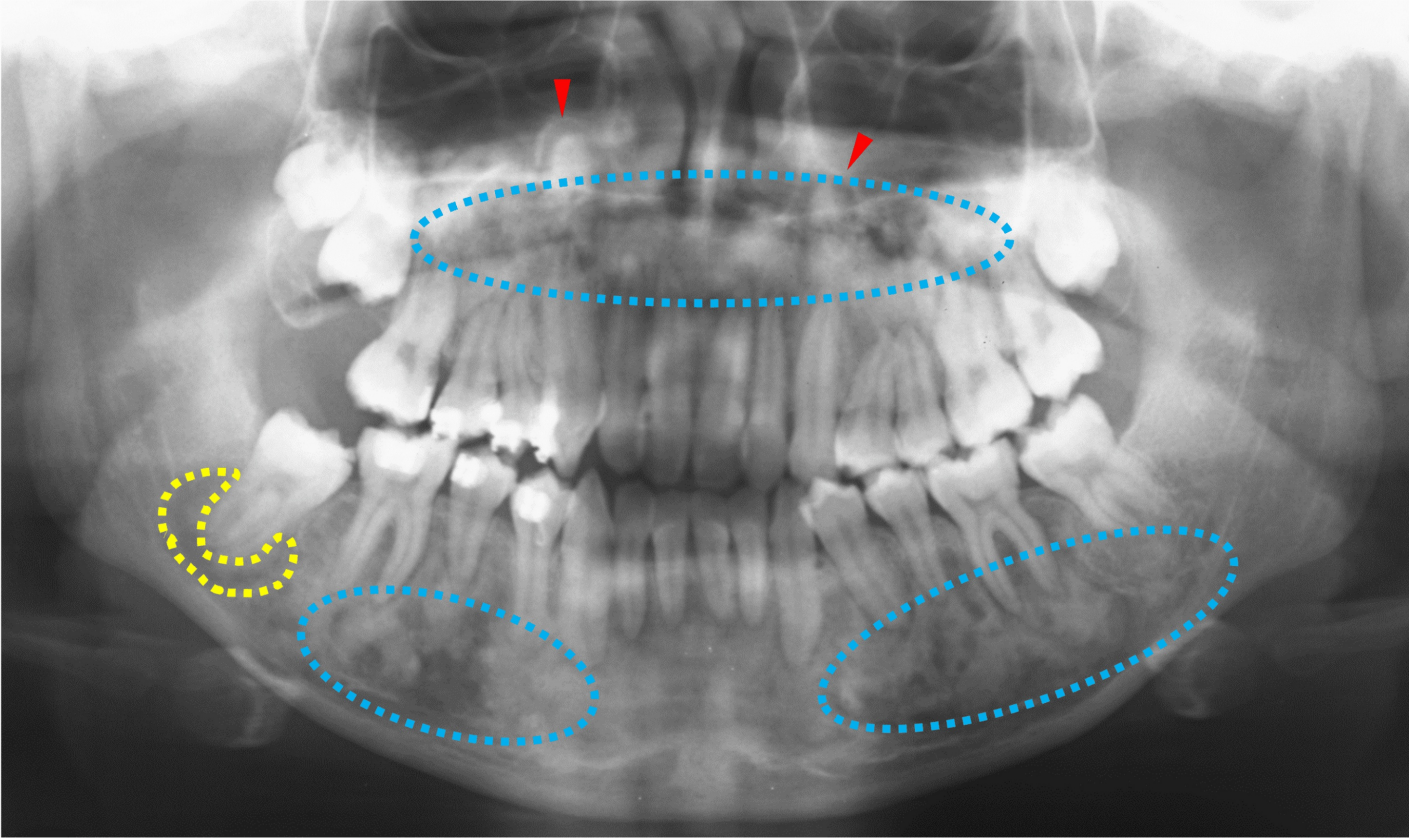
Maxillofacial radiograph at age 15 Blue dotted circles indicate the lesions in both the upper and lower jaws with enlarged frosted shadows. Red arrows indicate stenosis of the maxillary sinus. The yellow dotted circle indicates a radiolucent lesion adjacent to the right mandibular second molar root
